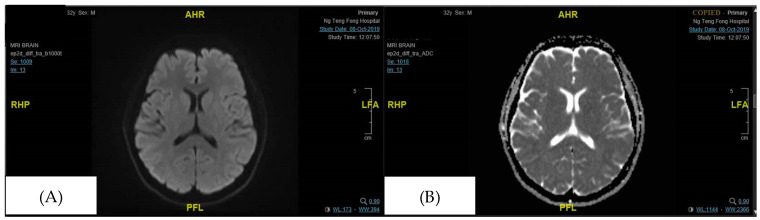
The focus of DWI signal abnormality in the splenium of the corpus callosum is no longer seen (in the follow-up visit 3 months later). (A) DWI (Diffusion Weighted Imaging), (B) ADC (Apparent Diffusion Coefficient).
